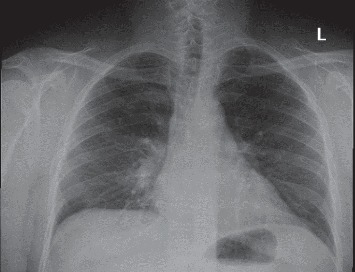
Chest X-ray with an anterior-posterior view: mild fullness in the right perihilar region.
